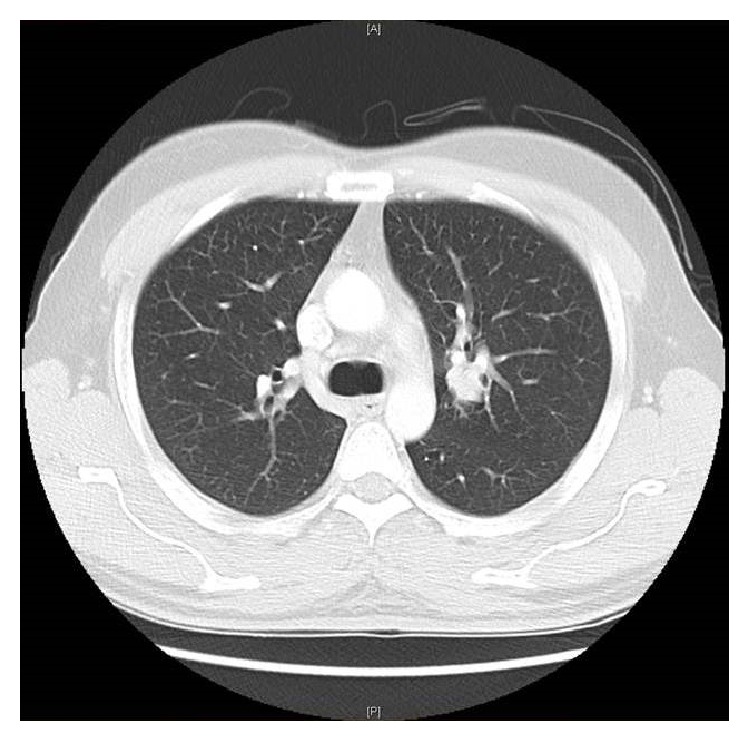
Follow-up CT chest redemonstrated the same lobulated round mass in the left upper lobe which is almost stable from the previous study. The mass is surrounded by ground glass opacity which is almost stable from the previous study.
